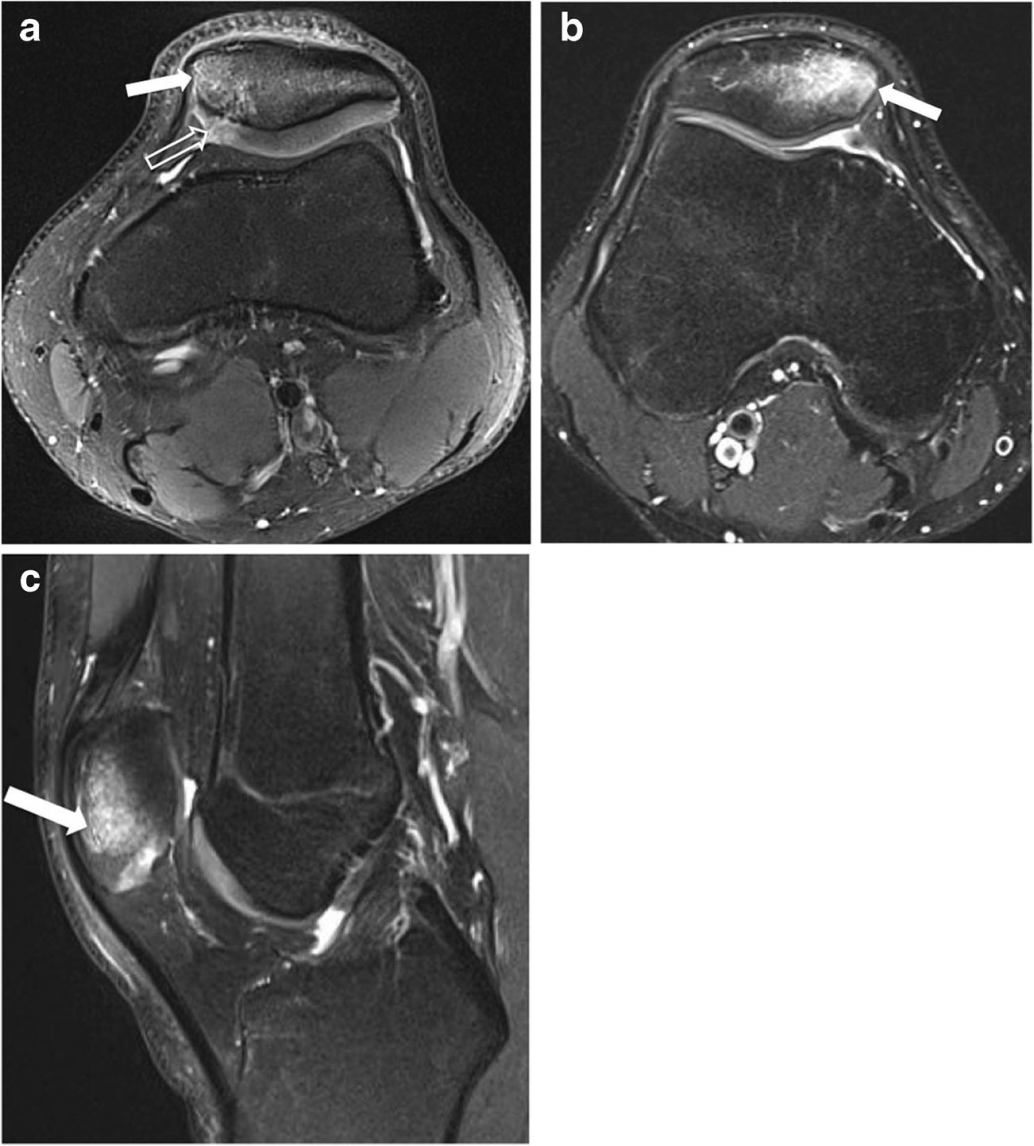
Examples of patellar contusions. (a) Axial fat-suppressed proton density-weighted MR image in a 20-year-old man shows hyperintense signal of the medial patellar bone marrow (*solid arrow*) and a fracture line through the adjacent cartilage (*open arrow*). Conventional radiographs were negative for fracture (not shown). (b) Axial and (c) sagittal fat-suppressed proton density MR images in another 20-year-old man following direct trauma, revealing bone marrow oedema (*arrow*) without cartilage damage. Conventional radiographs were also negative for fracture (not shown)
